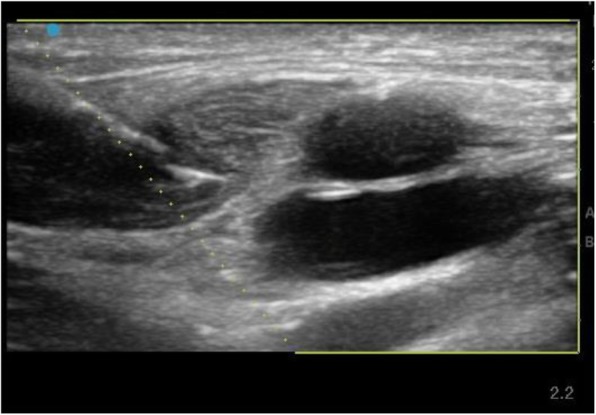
Ultrasound image showing the needle path and vessels in the oblique approach
